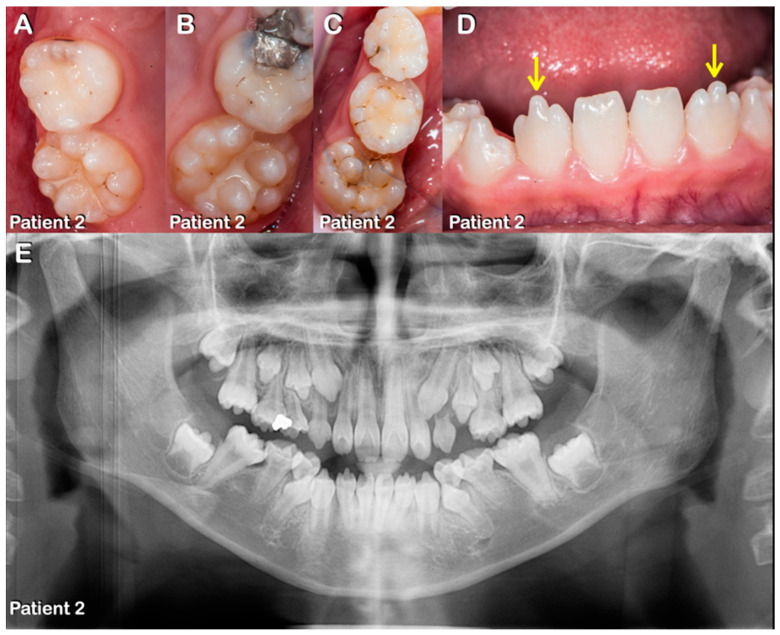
Patient 2. (A–E) Mixed dentition. (A–C) Round-shaped primary and permanent molars with multiple supernumerary cusps. (D) Prominent medial mamelon of the mandibular lateral permanent incisors (arrows). (E) Panoramic radiograph showing agenesis of all third permanent molars, single-rooted primary and permanent molars, and taurodontism.
